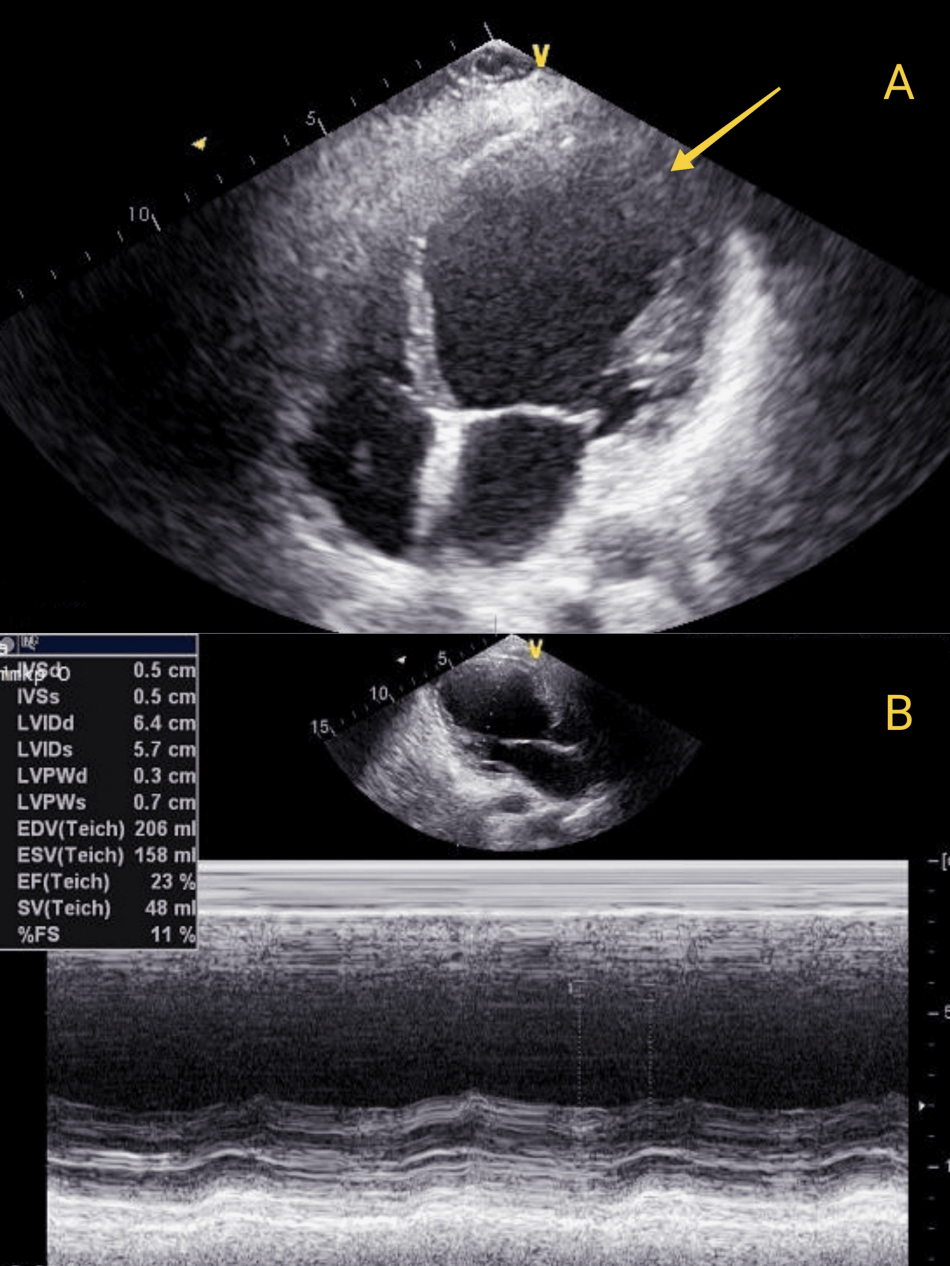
Cardiac echography A: apical 4 chamber view (arrow: dilated left ventricle); B: M-mode left ventricle measurements
